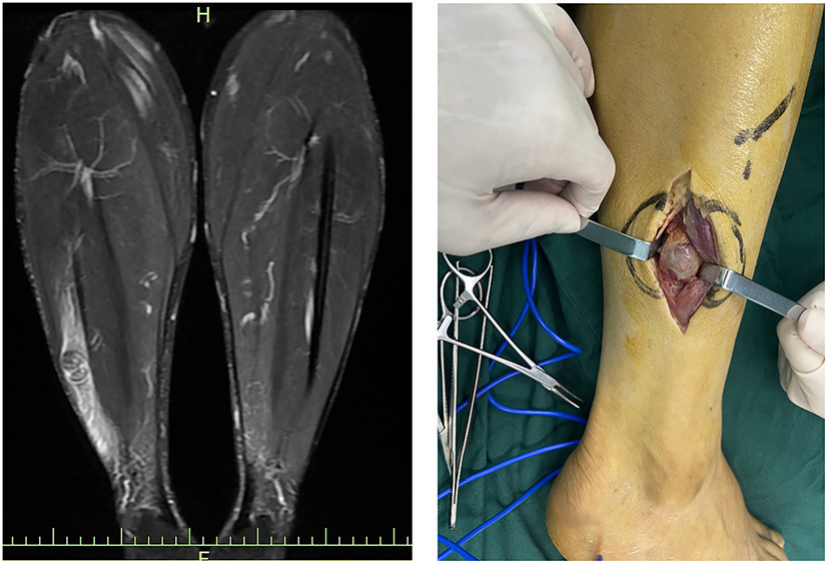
MRI shows that the tumor is located between the peroneus longus muscle.
